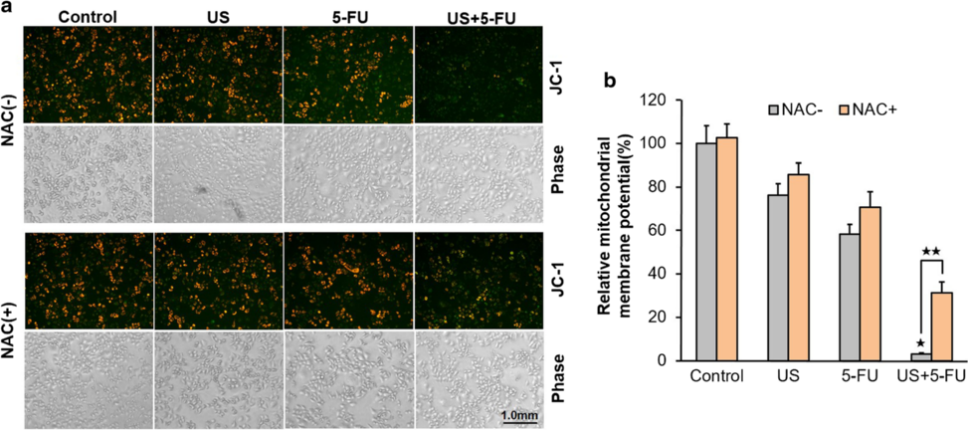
Alteration of mitochondrial membrane potential by the treatment of low-intensity ultrasound combined with 5-FU. a Representative mitochondrial membrane potential (Δψm) in BEL-7402 cells after the treatment. b The relative level of Δψm by the fluorescent intensity quantified with IOD values. Data was presented as the mean ± SD (*n* = 6, ^★^
